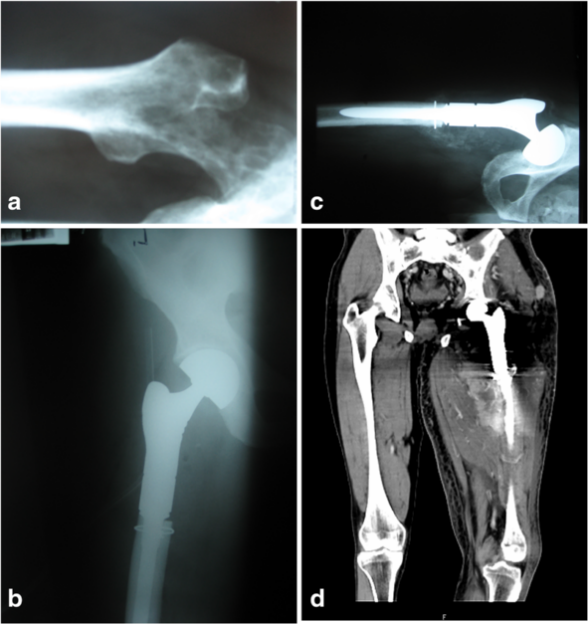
Chronologically arranged radiograms of kidney cancer metastases to the proximal femur (a) after resection of bone tumor and modular prosthesis implantation (b). Next, the radiogram (c) shows the postoperative recurrence of the disease. A coronal view from an MRI (d) shows a large tumor involving the whole thigh. There is visible damage to the femur and a loose prosthesis
